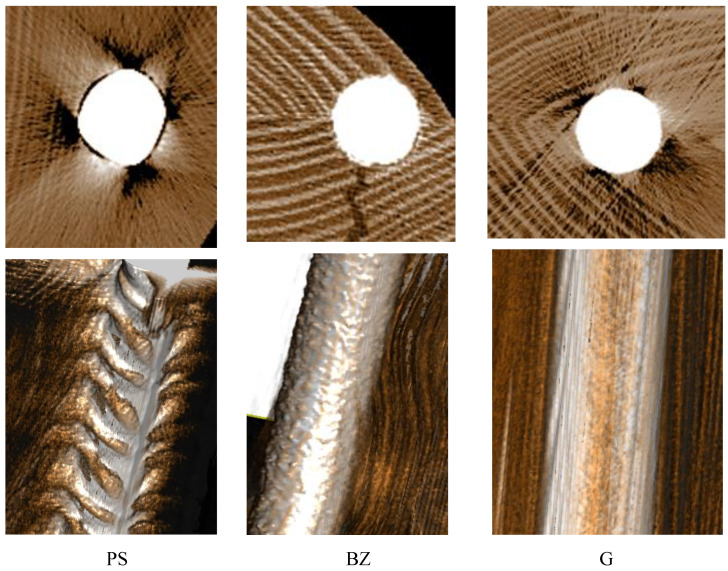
CT scans (1:1 scale). Upper image line—crosswise view, lower image line—longitudinal view.
